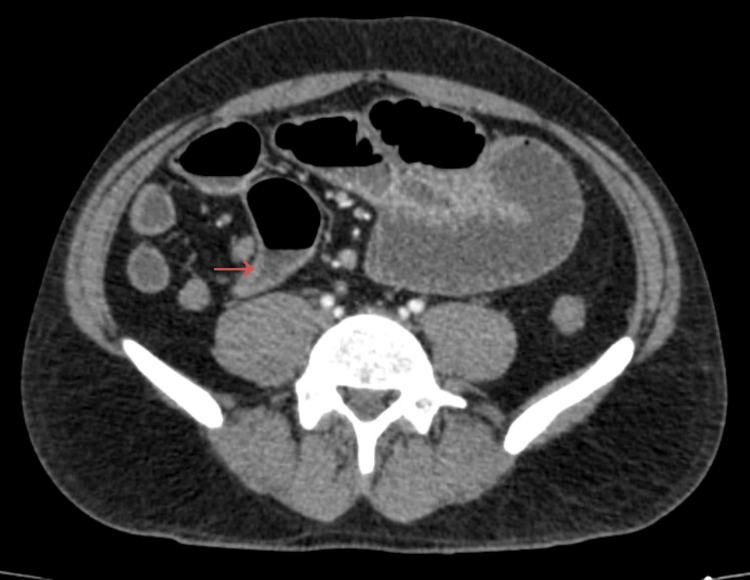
Axial contrast-enhanced CT showing Meckel’s diverticulum. Axial contrast-enhanced computed tomography (CT) image demonstrating a blind-ending, sac-like structure arising from the antimesenteric border of the terminal ileum (arrow), containing intraluminal material, consistent with Meckel’s diverticulum.
